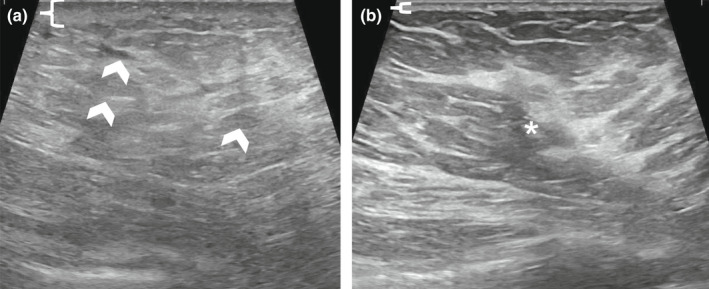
42‐year‐old woman with a history of breast conservation therapy performed 9 months prior presented to the emergency department with worsening right breast pain and redness despite being treated empirically with clindamycin. In the upper outer right breast at the site of clinical concern, ultrasound was performed with an L3‐12 MHz transducer with harmonics and the images were captured from the skin to the chest wall. Targeted ultrasound demonstrated skin thickening measuring 6 mm (bracket) and underlying oedema manifested as subtly increased echogenicity (chevrons), but no drainable fluid collection (a). A comparison with the contralateral (left) breast was performed, which showed normal skin thickness measuring 1 mm (bracket) and normal heterogeneously dense fibroglandular tissue (asterisk) (b). Imaging findings are consistent with right breast mastitis and overlying cellulitis without an underlying abscess. Clinical follow‐up of the right breast erythema and pain with repeat targeted imaging in the setting of worsening symptoms was recommended. Fortunately, this patient's symptoms were fully resolved, and she did not require additional targeted imaging.
